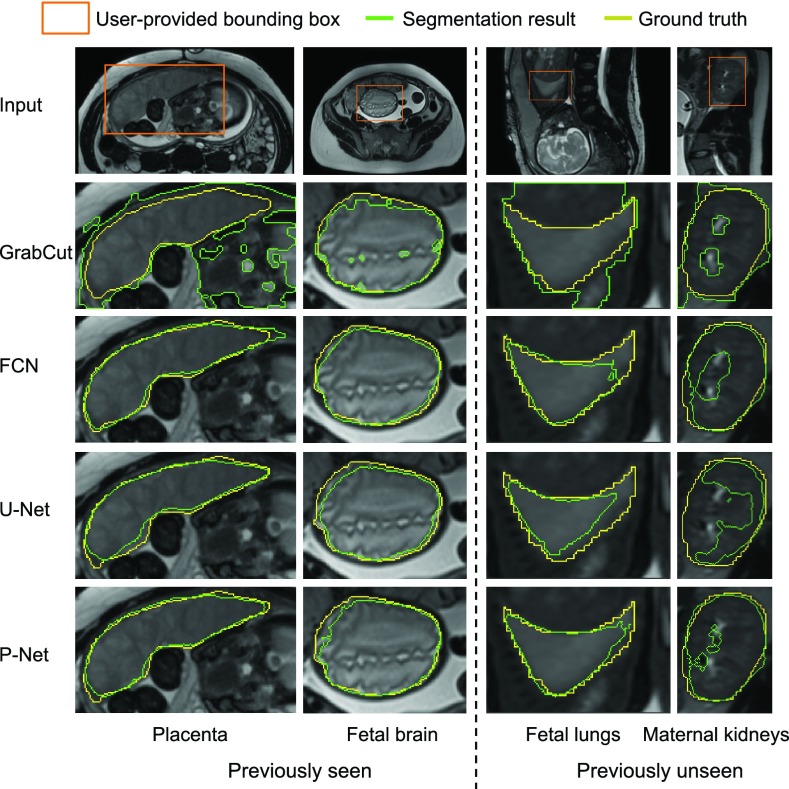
Visual comparison of initial segmentation of multiple organs from fetal MRI with a bounding box. All the methods use the same bounding box for each test instance. Note that fetal lungs and maternal kidneys are previously unseen objects but P-Net works well on them.
